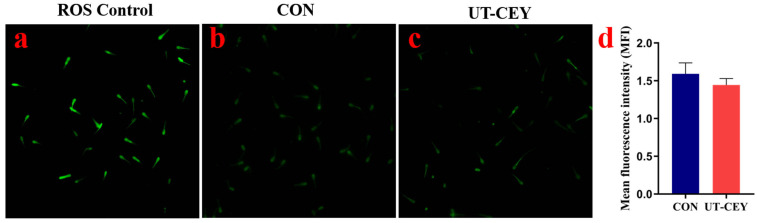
Effect of ultrasonicated chicken egg yolk on ROS levels in post-thaw boar sperm. (a–c) show ROS fluorescence images of the positive control group, CON, and UT-CEY, respectively; (d) shows the mean fluorescence intensity of ROS in sperm cells.
